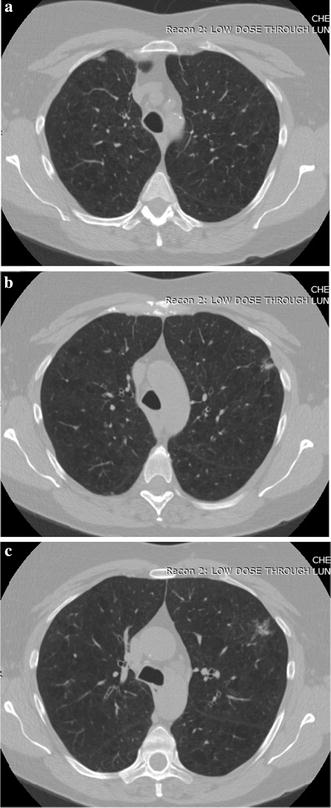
Original CT frames. a Frame 117, b Frame 138, c Frame 159
